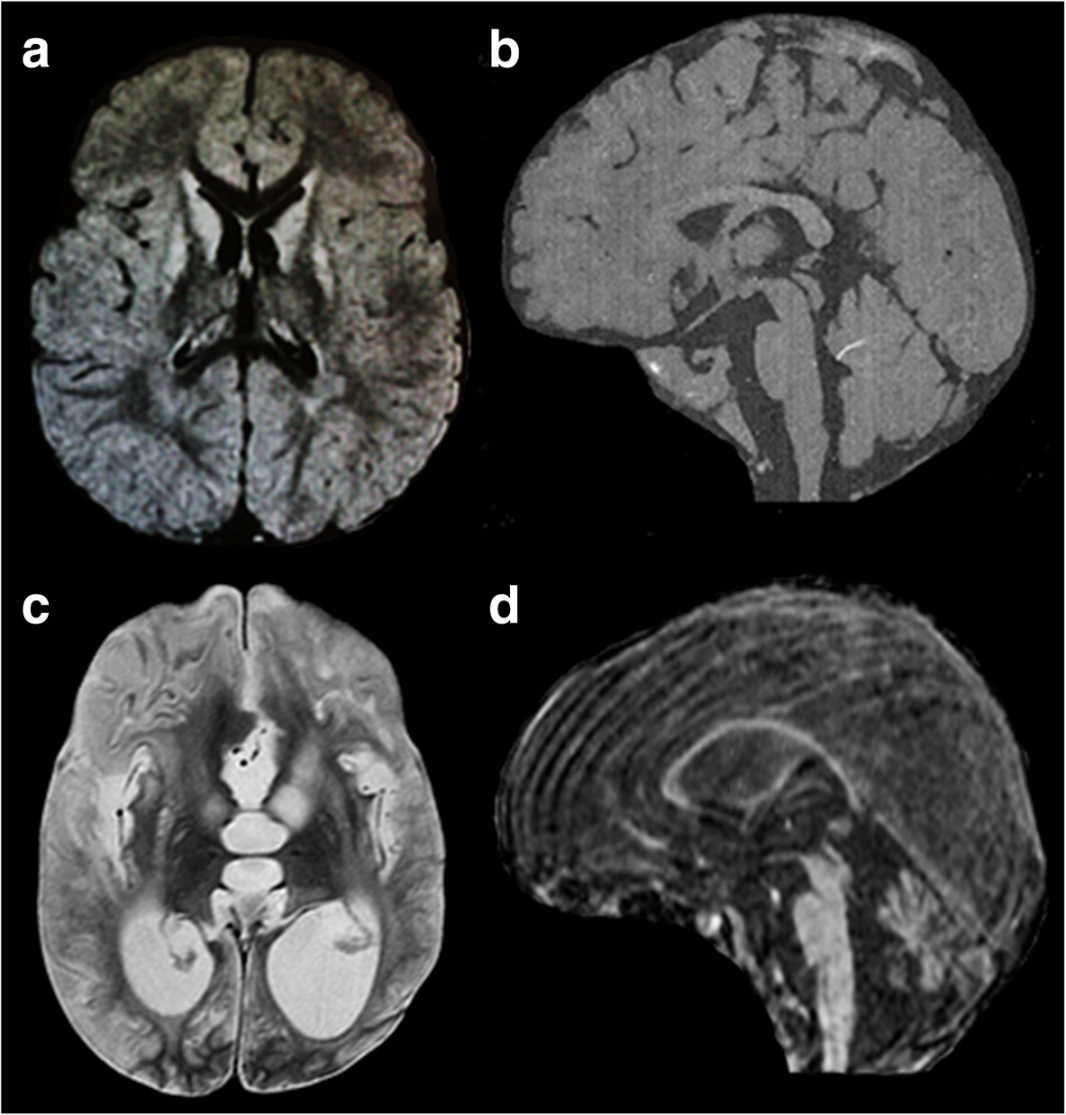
1.5 T low-quality images of the first MRI examination of the boy (16 months); the best available images were chosen. Axial FLAIR (a) slice shows the typical pattern of LS with basal ganglia involvement (caudate and lenticular nuclei) and mild involvement of thalami bilaterally. Sagittal T1 slice (b) shows normal tropism of the corpus callosum and cerebellum. Last MRI examination at 12 yrs. (c-d) shows marked progression of basal ganglia involvement with enlargement of lateral and third ventricles, indicating global atrophy. Sagittal FLAIR slice (d) highlights marked thinning of the corpus callosum, a consequence of brain atrophy, and severe cerebellum atrophy. The presence of significant movement artifacts is related to MRI without anesthesia, because of critical conditions of the patient
